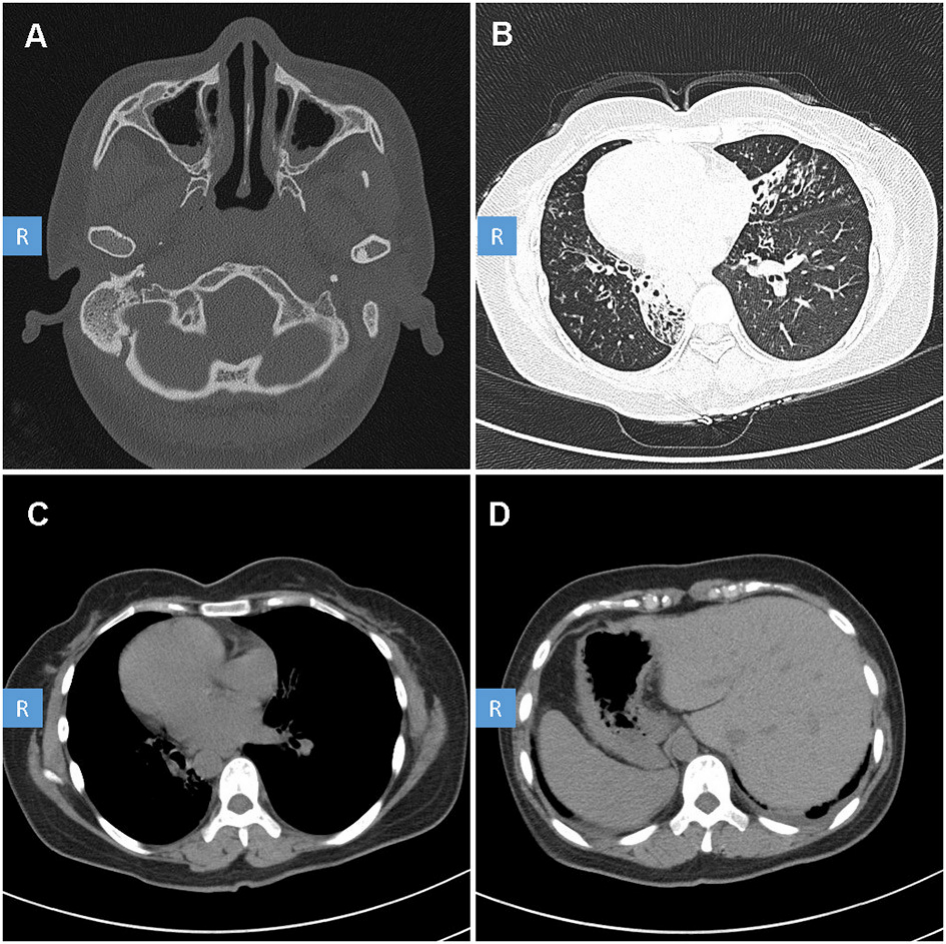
High-resolution computed tomography (HRCT) scan of the patient with PCD. CT scan showed: (A) Sinusitis. (B) Diffuse bronchiectasis. (C) Right-side heart. (D) Abdominal organ heterotaxy: liver in the left side, stomach and spleen in the right side.
